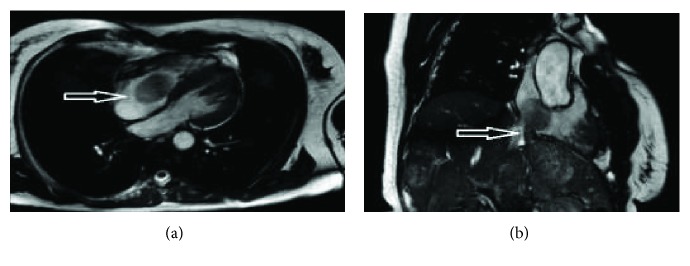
Cardiac MRI confirms the mass in (a) the right atrium with prolapsing through the tricuspid valve and (b) the contiguous extension of the liver metastasis to the IVC and right atrium (white arrows).
